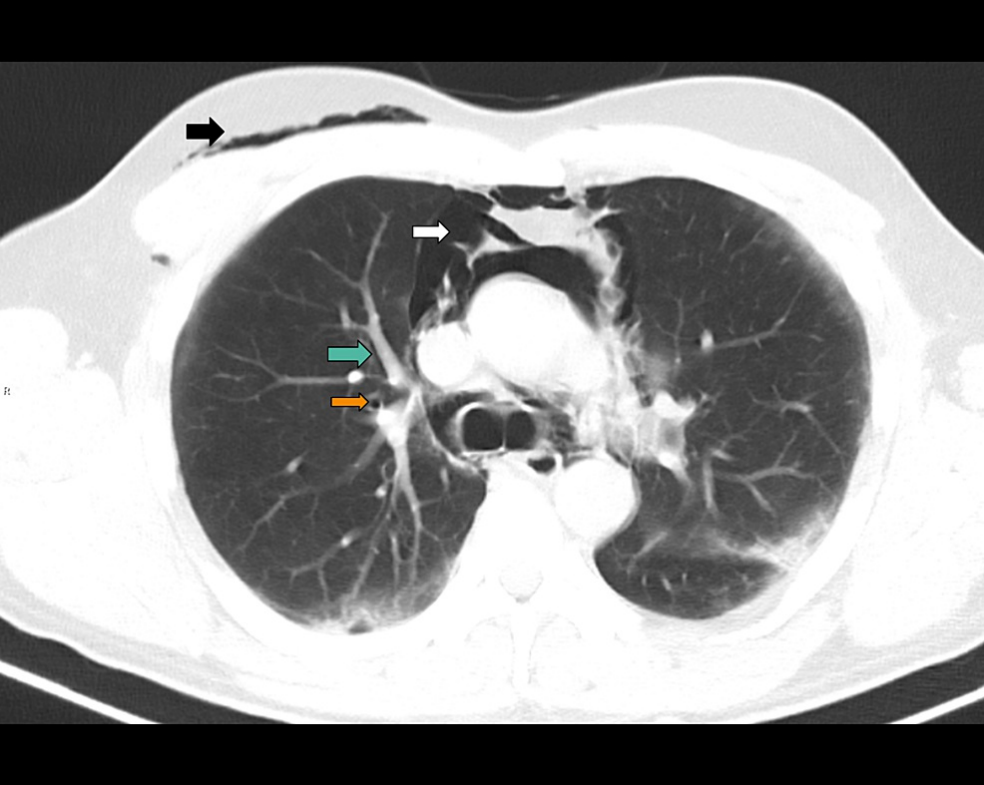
CT scan of the chest from day 3: axial slice showing air around bronchovascular segment aka the Macklin effect (green arrow), pneumomediastinum (white arrow), cystic air space (orange arrow), and subcutaneous emphysema (black arrow).
